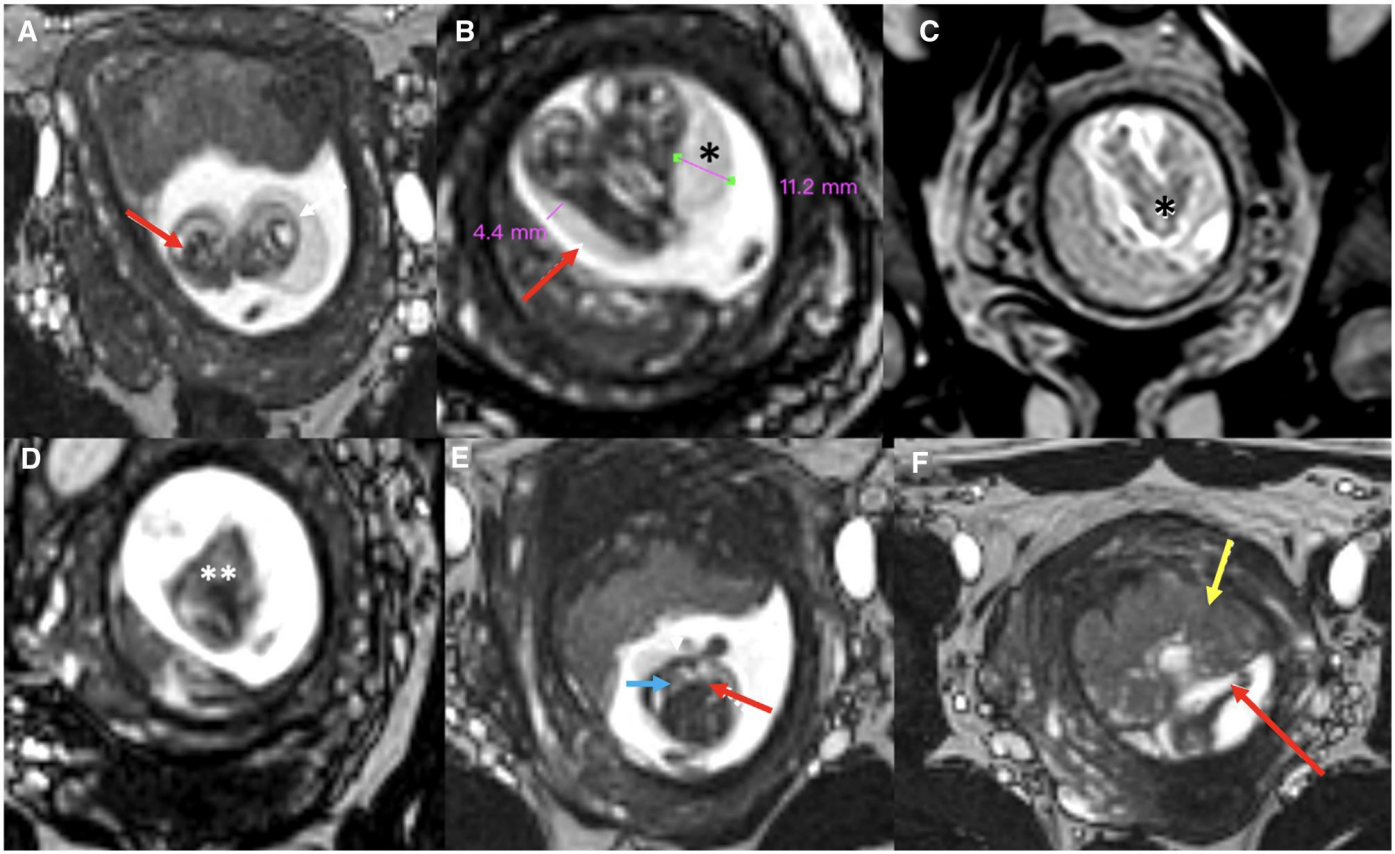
BTFE (Balanced Turbo Field Echo T2-weighted gradient echo sequence) foetal MRI. Sagittal images showed separate foetal heads (red arrows in A) and separate necks (B) with increased NT (nuchal translucency) (red arrow) and cystic hygroma (* in B), with fusion involving thorax (denoted by * in C) and abdomen (denoted by  in D); (E) coronal image showed single heart (blue arrow) and liver (red arrow); (F) anteriorly located placenta (yellow arrow) and normal insertion site of single umbilical cord (red arrow).
